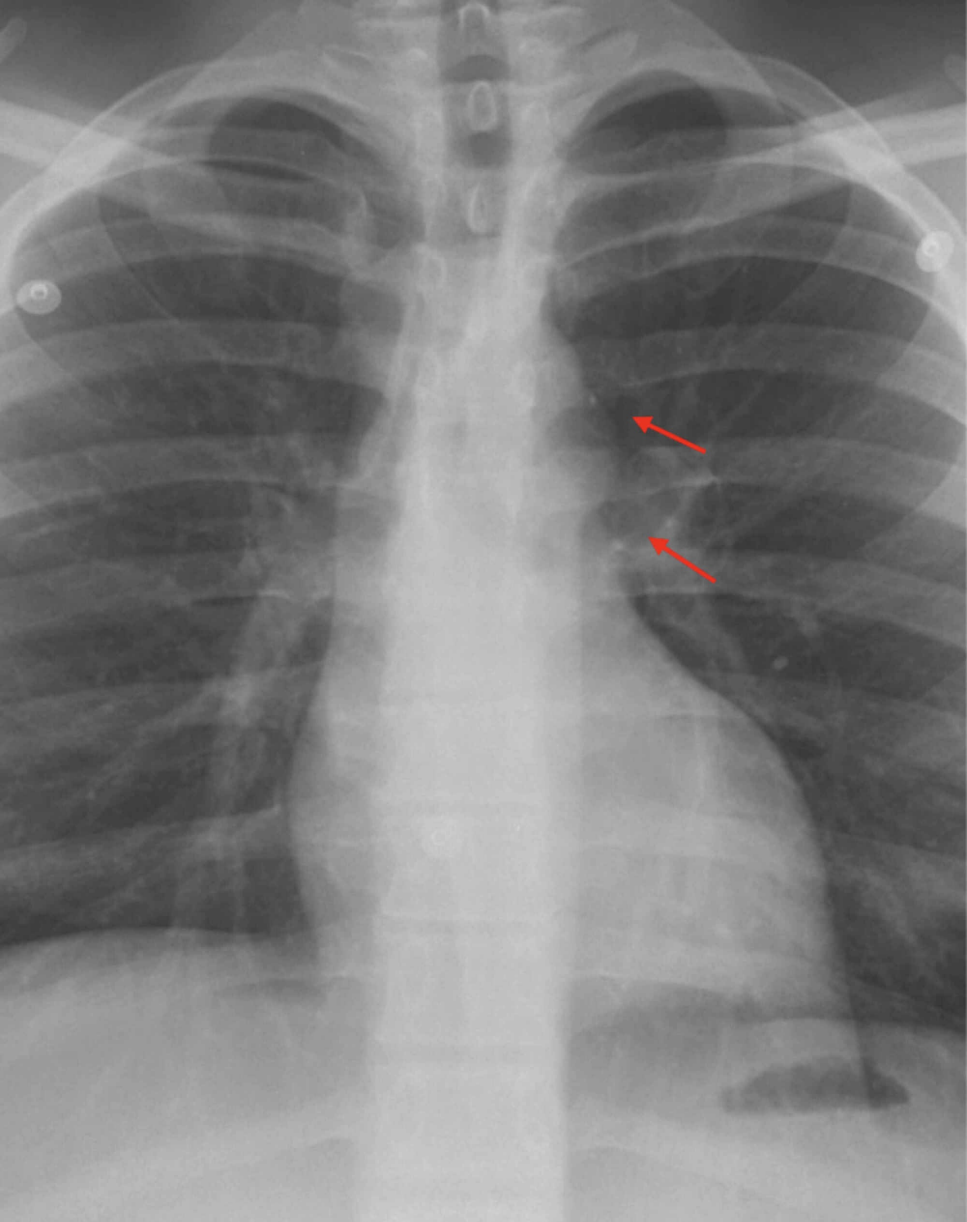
Chest X-ray revealing wide mediastinum (red arrows)
